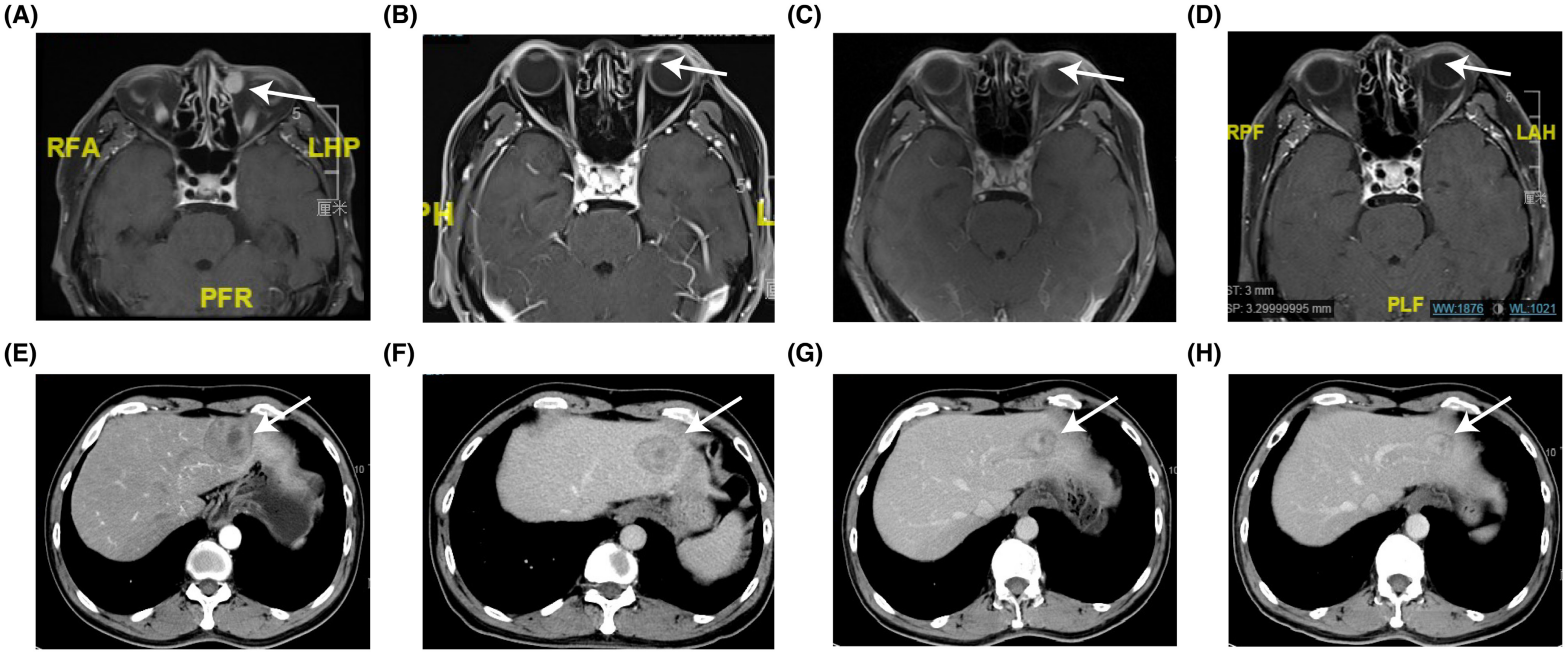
One patient with metastatic lacrimal sac melanoma who achieved partial response after pembrolizumab plus Rh‐endostatin therapy. MRI images of lacrimal sac melanoma before treatment (A), 3 months (B), 5 months (C) and 14 months (D) after treatment. CT images of liver metastases at baseline (E), 3 months (F), 5 months (G) and 7 months (H) after treatment.
